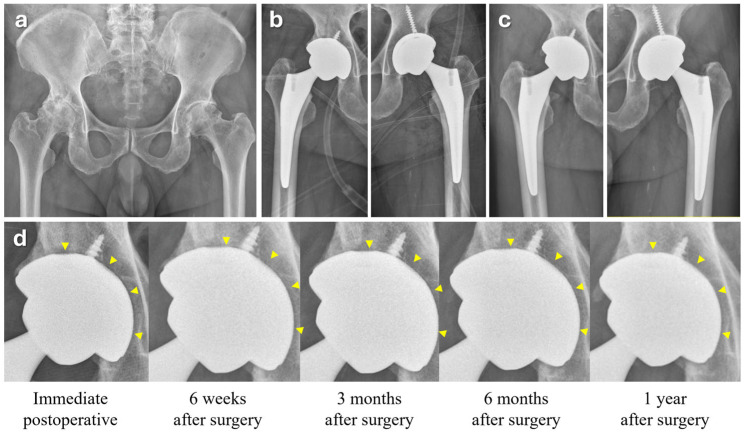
Serial radiographs following total hip arthroplasty using a 3D-printed acetabular component. (a) Preoperative hip AP radiograph showing bilateral advanced osteoarthritis in the hip joints. (b,c) Serial AP radiographs of the operated hips from immediately after surgery to 4 years postoperatively. (d) Magnified views of the acetabular component at each time point. Yellow arrowheads indicate the radiolucent line between the cup and the host bone. The gap observed immediately after surgery gradually decreased over time, becoming undetectable from 6 months postoperatively, suggesting progressive osseointegration of the implant.
